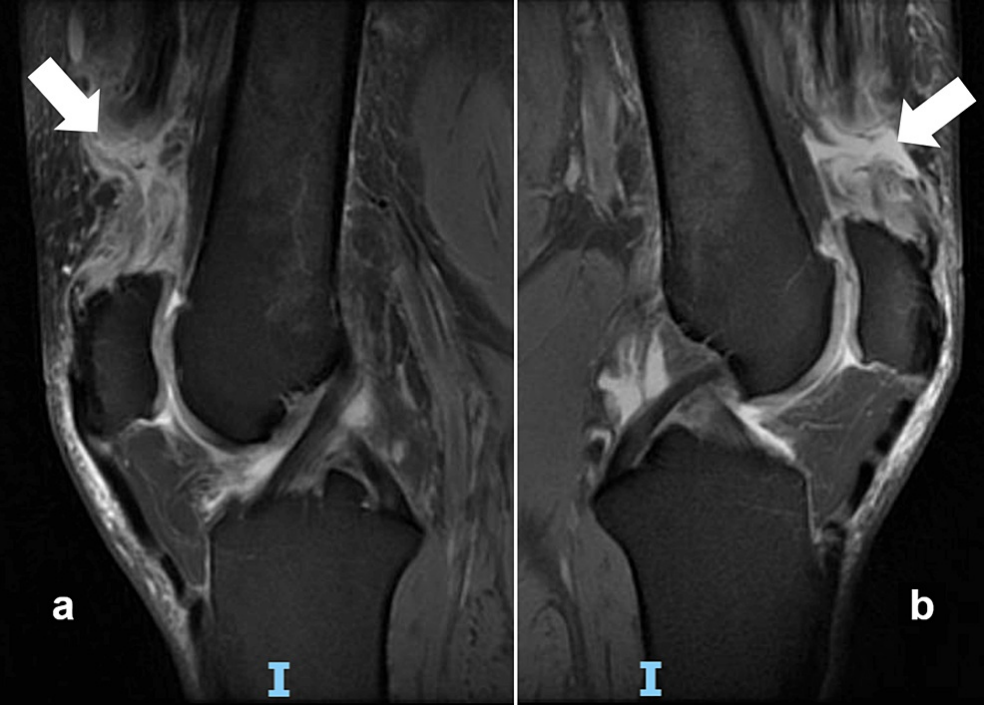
T2 MRI sagittal cuts of the right (a) and left (b) knees. White arrows indicate the site of rupture for each quadriceps tendon.
